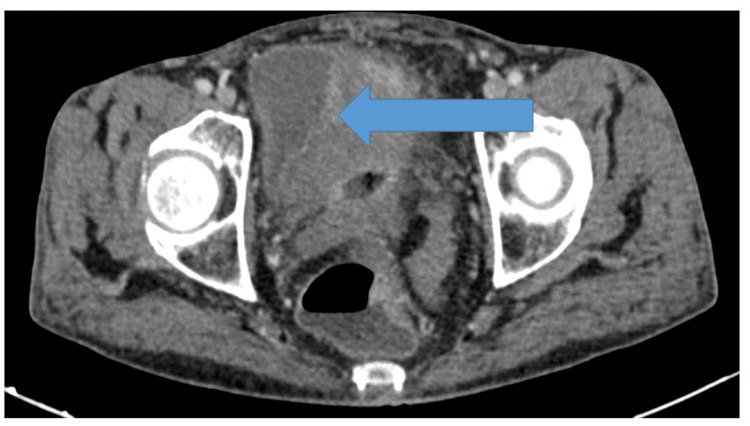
Axial CT scan showing a supravesical inflammatory collection in contact with the bladder dome, with associated bladder wall thickening.
